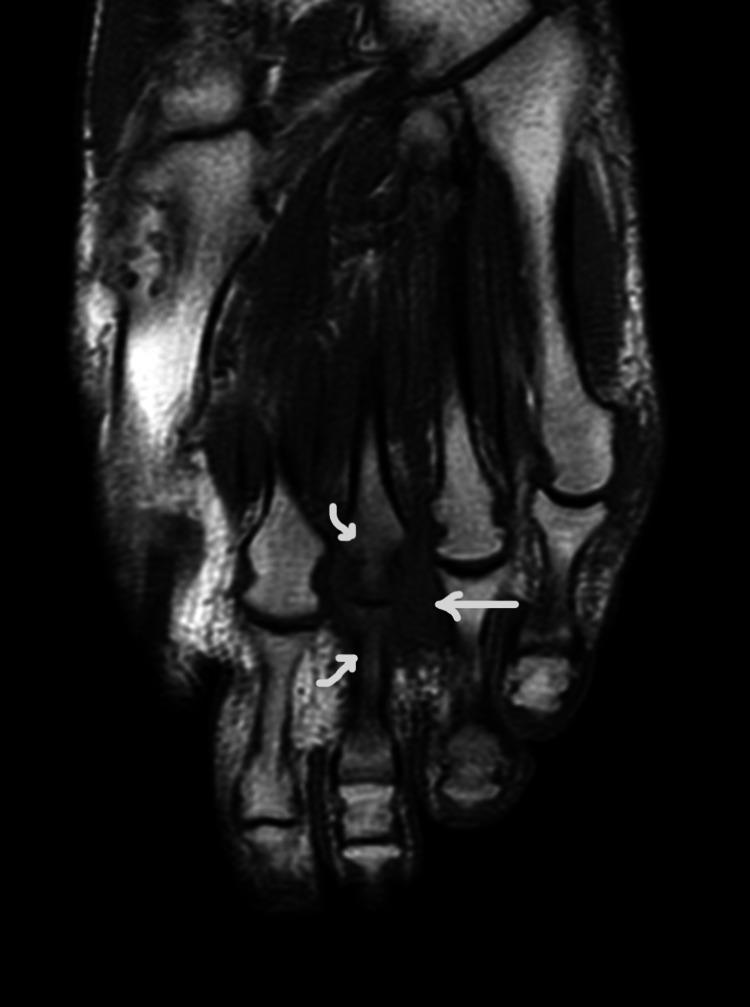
T2-weighted long-axis left-foot magnetic resonance imaging showing marked bone edema of the third metatarsal bone and the third proximal phalanx, and heterogeneous signal widening of the third intermetatarsal space.
